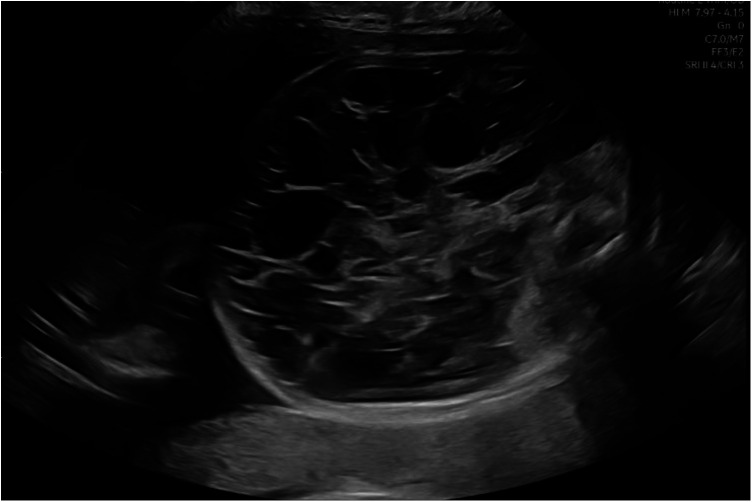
Ultrasound appearance of the lesion at 30 weeks of gestation on an axial scan of the fetal abdomen (Bambino Gesù Children's Hospital, Rome, Italy).
